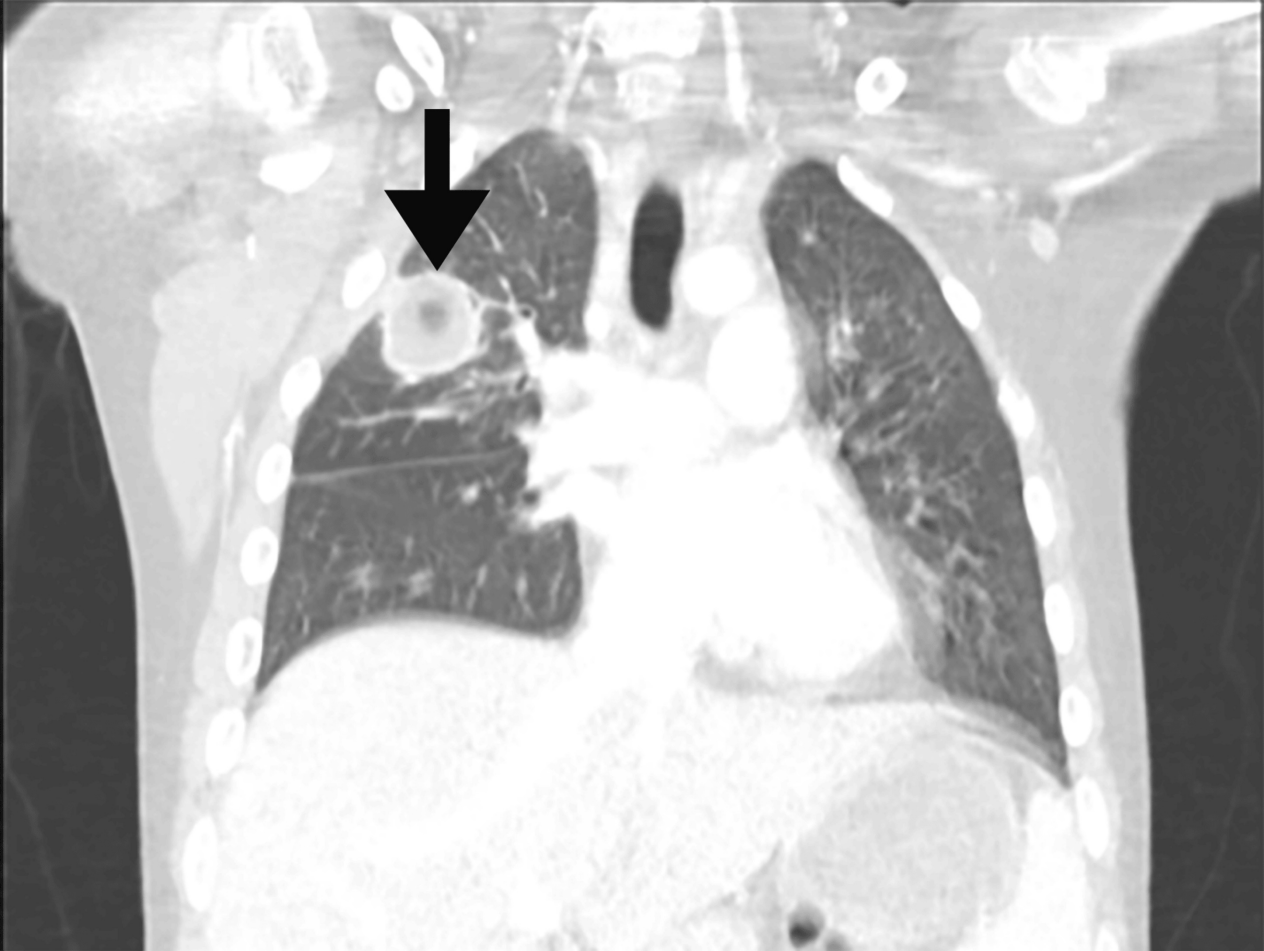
Computed tomography image of right upper lobe cavitary lesion
